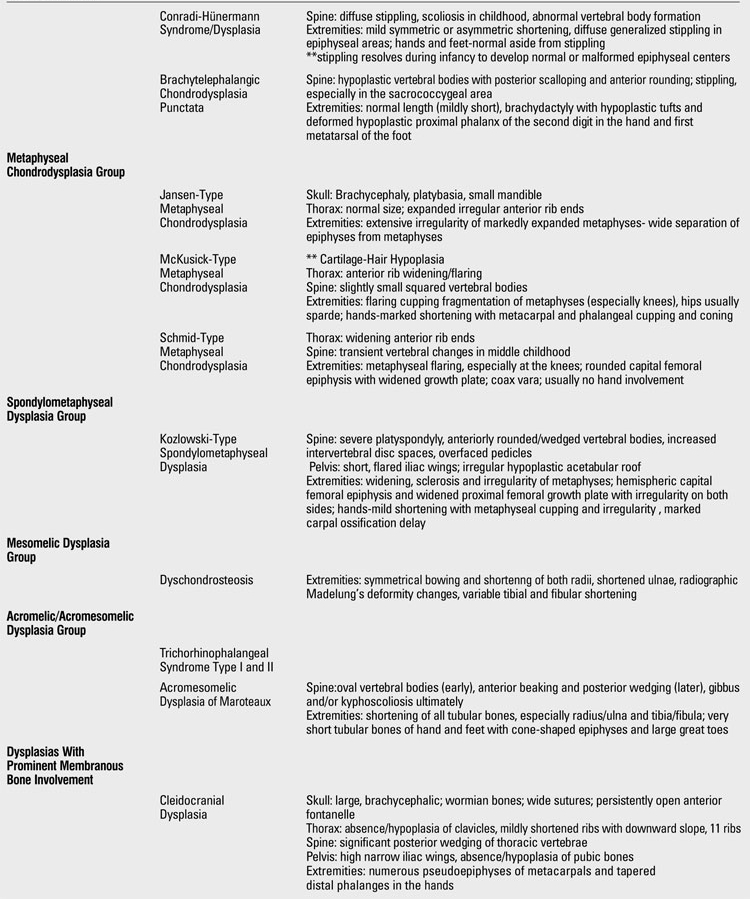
Clues for radiographic diagnosis of skeletal dysplasias
